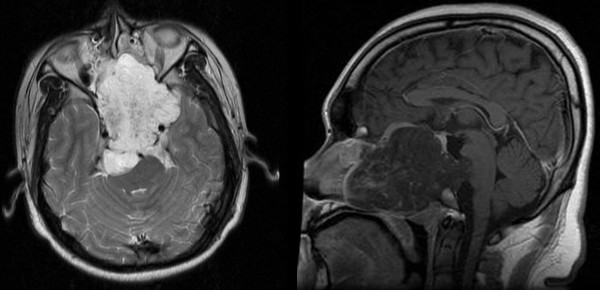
Sagittal and axial views of brain MRI scan. Image shows tumour in the nasopharynx extending from nasal cavity to brainstem posteriorly.
